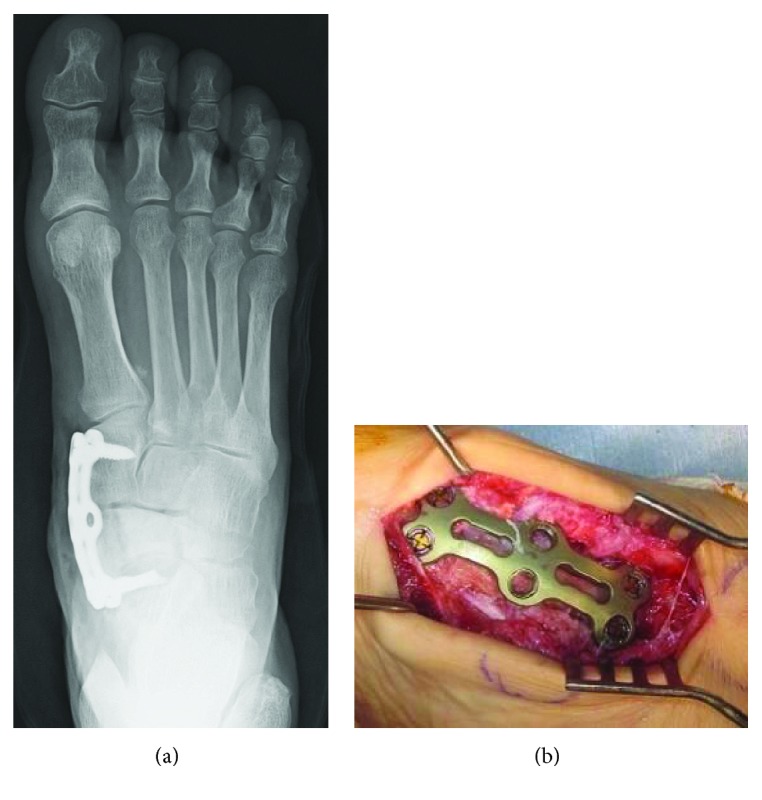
(a) Arthrodeses of the talonavicular and naviculocuneiform joints using CSLP-VA. (b) Postoperative radiograph shows fixation using CSLP-VA with 4 4.0 mm locking screws.
